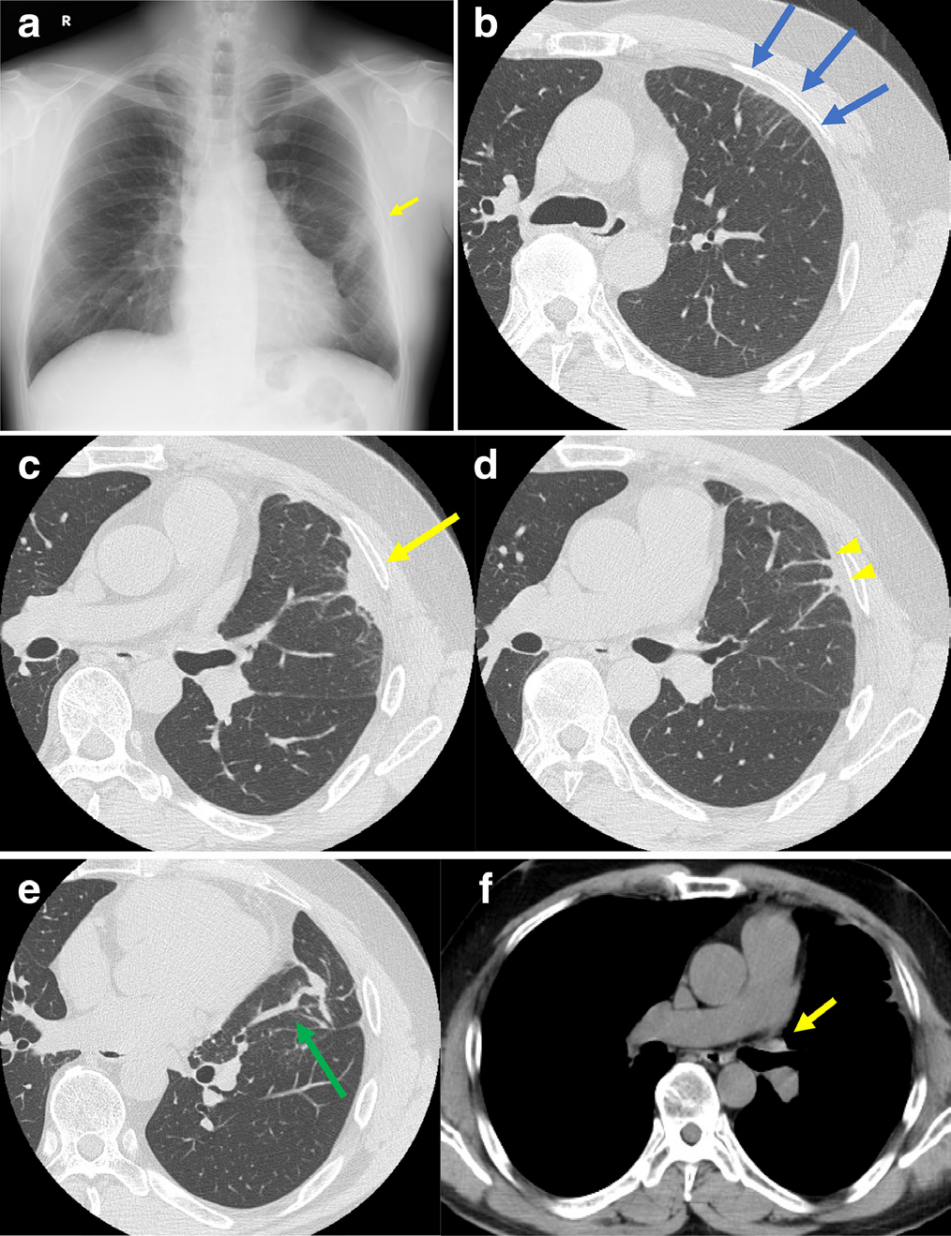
1 year and 3 months after second catheter ablation therapy (A) Chest radiograph demonstrated the consolidation in the left middle lung zone (B–E) CT revealed linear opacities (blue arrow), parenchymal bands (yellow arrow head), peripheral consolidation (yellow arrow) and the dilatated and tortuous pulmonary vein (green arrow) in the anterior and lingular segment of the LUL. The left major fissure was incomplete lobulation. (F) Plain CT images brought the stenosis of the left upper PV (yellow arrow) near the atrial junction. LUL, left upper lobe; PV, pulmonary vein.
